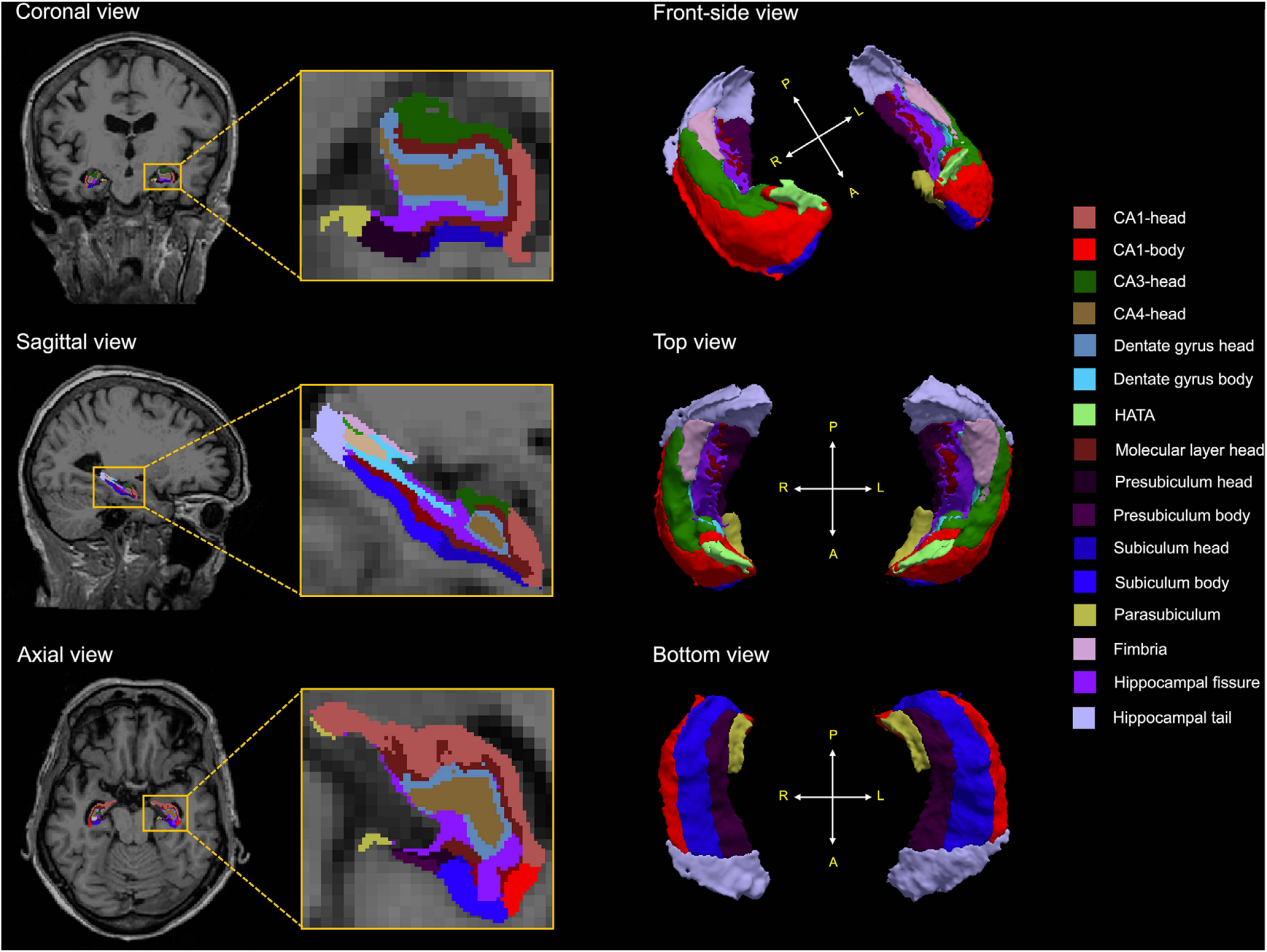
Hippocampal subfield segmentation in two‐dimensional and three‐dimensional views. The left panel shows representative coronal, sagittal, and axial slices with hippocampal subfields overlayed on T1‐weighted magnetic resonance images. The right panels present three‐dimensional surface renderings of the hippocampal subfields from the front, top, and bottom perspectives. The color coding corresponds to individual subfields, as shown in the legend. P, posterior; A, anterior; R, right; L, left; CA, cornu ammonis; HATA, hippocampal‐amygdala transition area.
